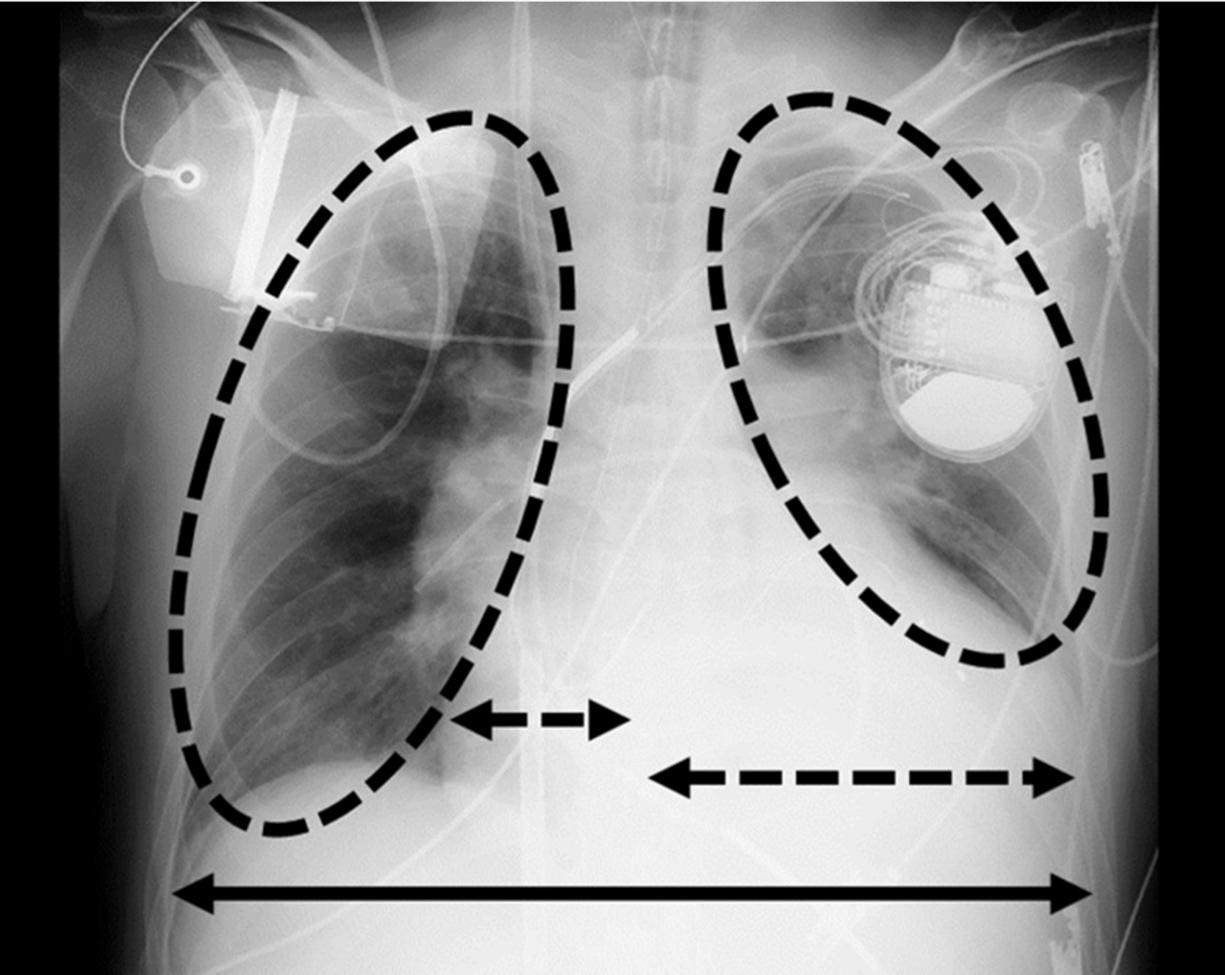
Frontal view of the chest X-ray on admission to our hospital (anteroposterior supine view). The right and left lung fields (circular dashed lines) showed reduced permeability. The cardiothoracic ratio calculated from the maximum transverse diameter of the lung fields (solid arrow) and the maximum transverse diameter of the heart (dashed arrow) in the figure was 66.6%.
